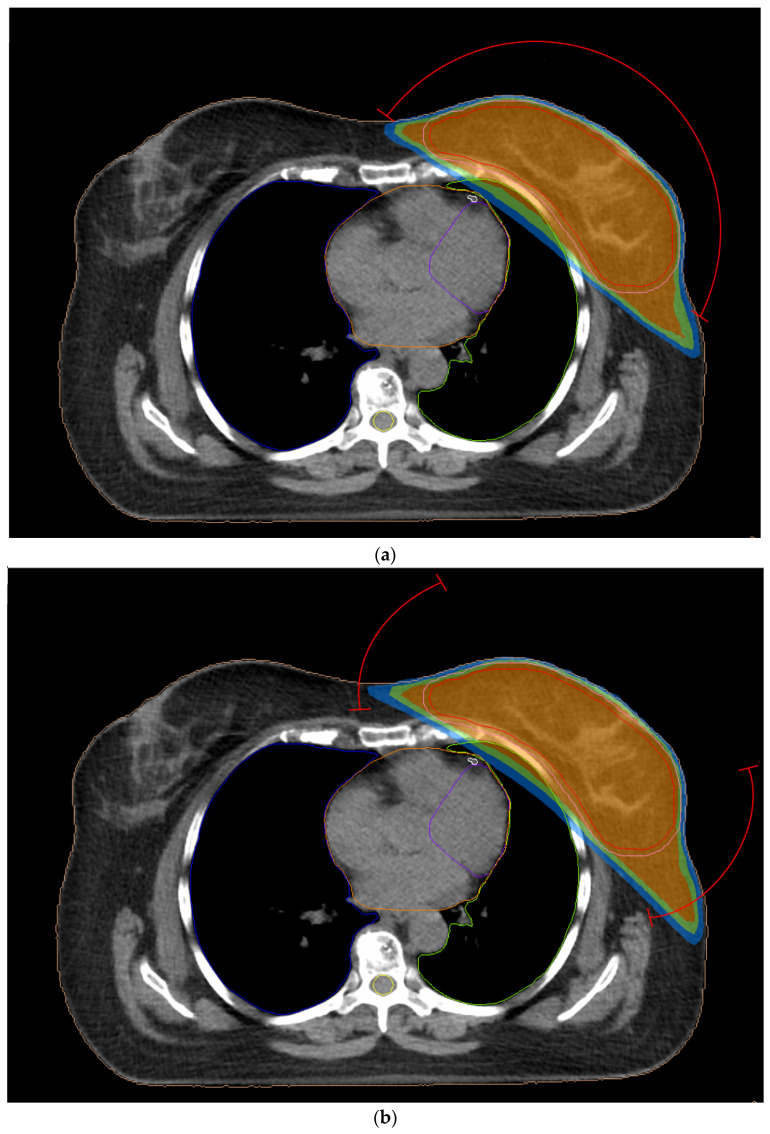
Computed tomography image with contoured structures: CTV (red); PTV (pink); heart (orange); ipsilateral lung (green); contralateral lung (blue); LAD (white); left ventricle (purple); spinal cord (yellow). Treatment plans of left breast cancer with dose distribution around the target volume (red) which treated with (a) two partial-arc VMAT and (b) four partial-arc VMAT. The isodoses of orange, green and blue color correspond to 95%, 85% and 70% of total dose, respectively.
